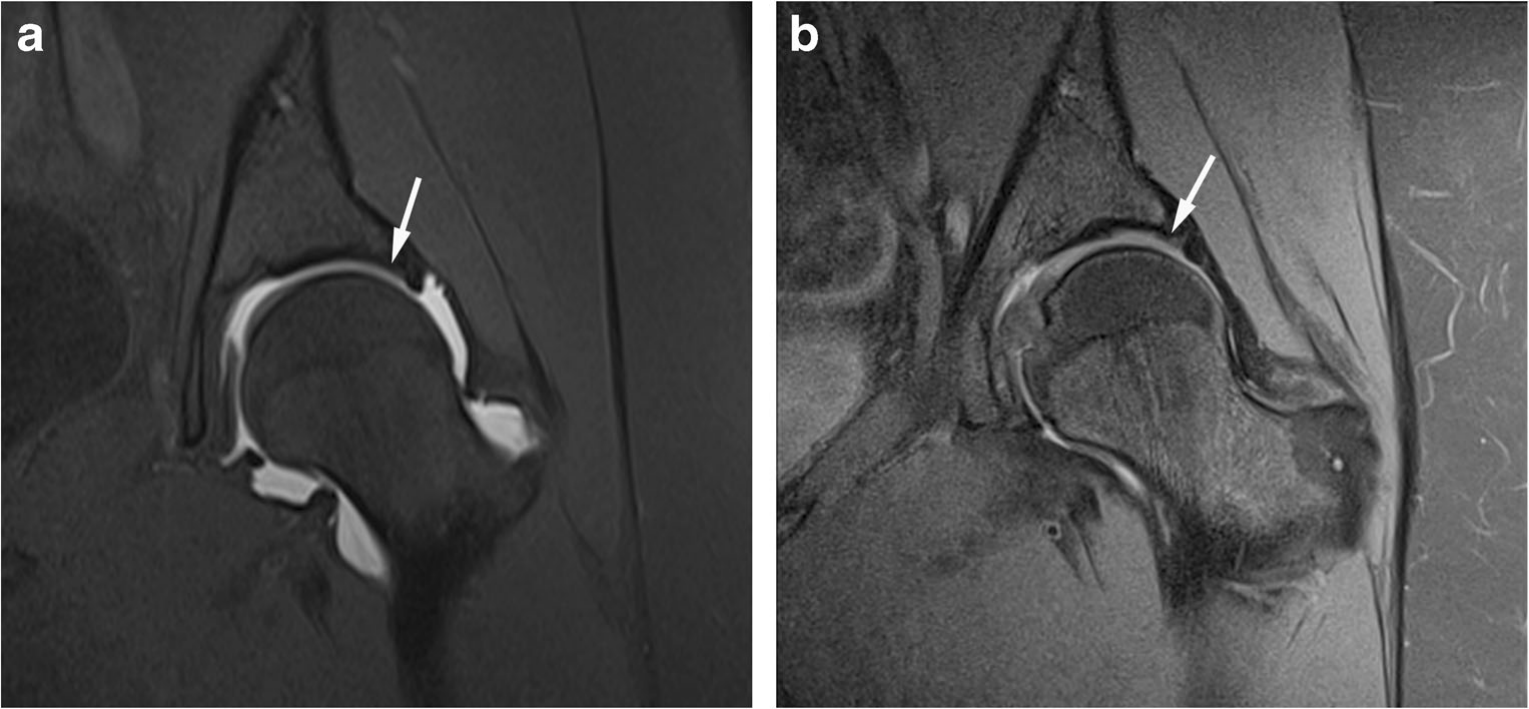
Superior acetabular cartilage delamination in a 31-year-old confirmed at surgery. Coronal (a) T1 FS 1.5T MRA image shows intact superior labrum and the adjacent cartilage was scored by both readers as normal. (b) PD FS 3T MR image shows intact superior labrum and the adjacent cartilage was scored by both readers as low T1 signal indicating delamination
